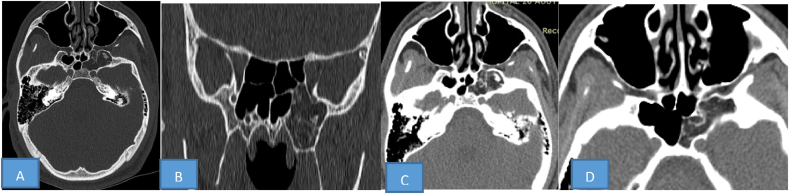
Brain CT-Scan: revealed: absence of aeration of the left sphenoidal sinus. Non-eroded, non-expansile lesion of fatty density lesion with a well-demarcated and internal curvilinear calcification in the location of left sphenoid sinus. The margin was osteosclerotic, and the adjacent bony structure was intact and showed no mass effect.
